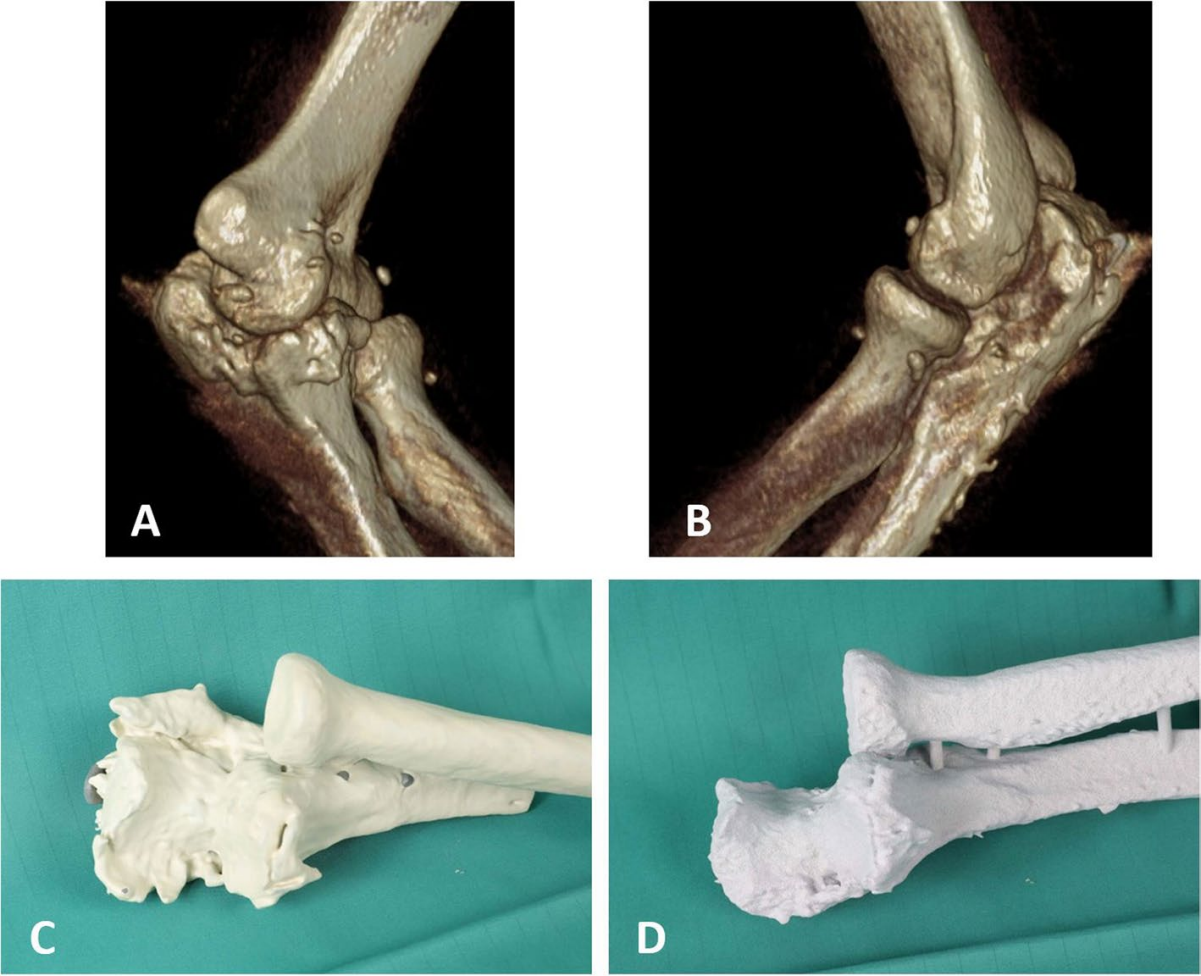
Postoperative CT scan (A, B) and 3D-model confirming the resection of all interfering osterophytes (C, D)
